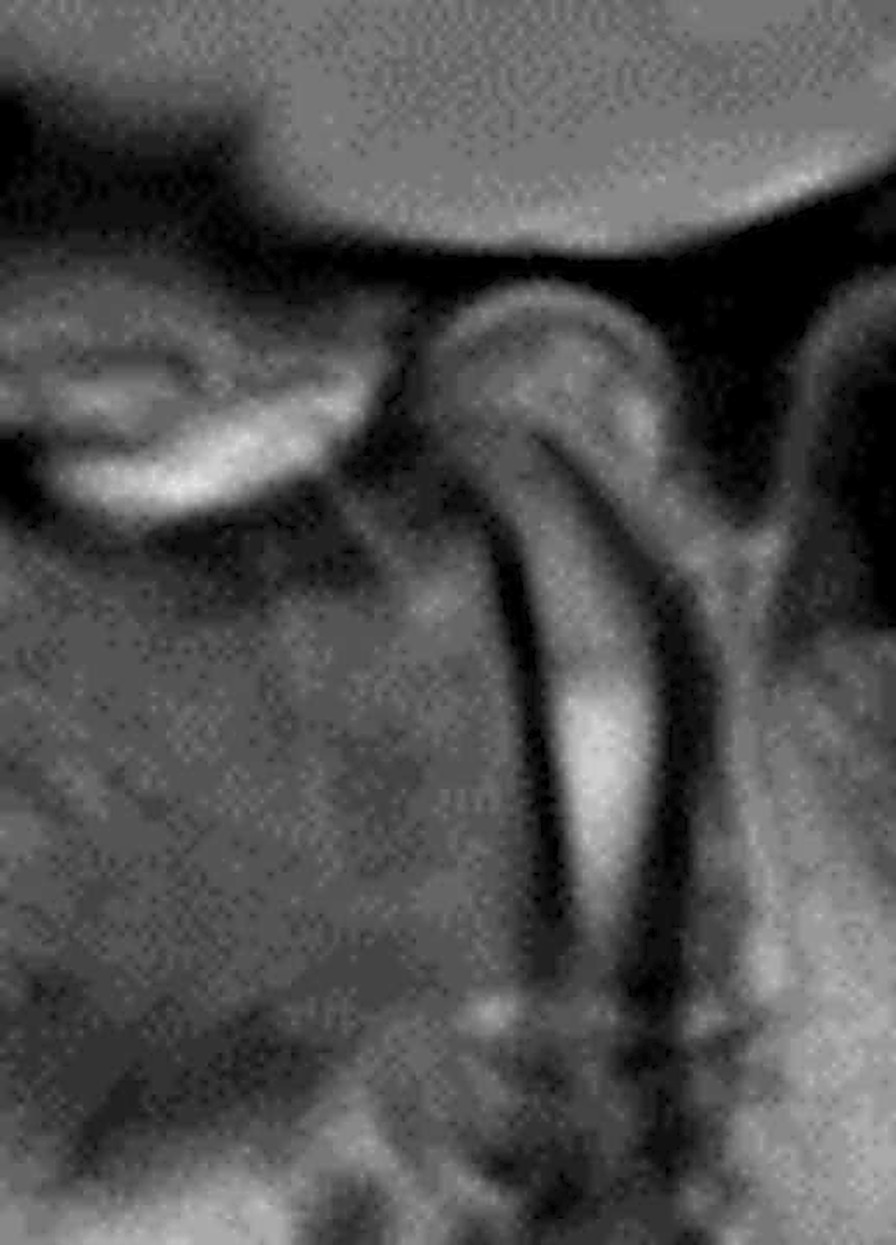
MRI open mouth right (12/19)
